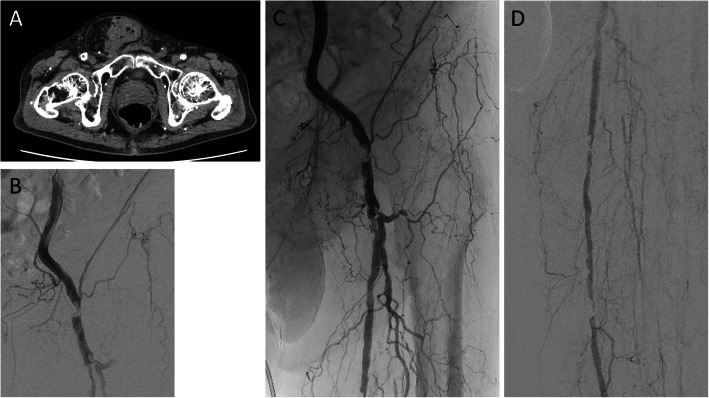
a Plain computed tomography scan shows a calcified plaque in the left CFA. b–d Control angiography shows total occlusion of the left CFA with a calcified plaque and total occlusion of the middle to distal SFA
